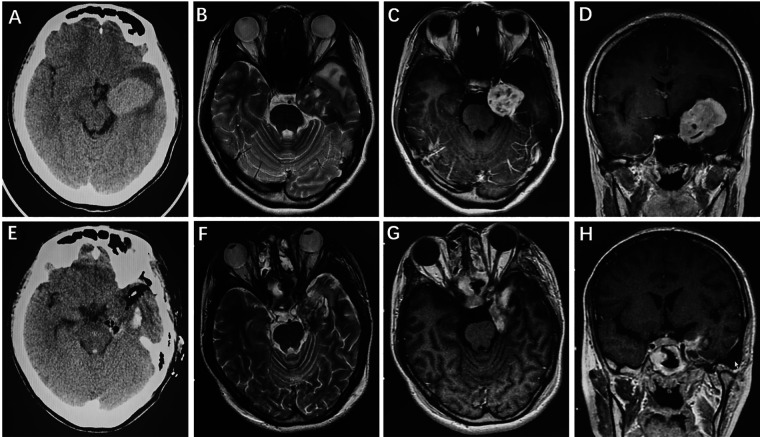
HPC invading the left CS and middle cranial fossa. Preoperative axial CT showed a slightly hyperintense lesion in the left CS and middle cranial fossa (A). Cranial axial MRI demonstrated isointensity on T2-weighted imaging (B). After enhancement, the axial and coronal MRI showed heterogeneous enhancement (C,D). Postoperative CT (E) and MRI (F–H) revealed that the tumor was subtotally removed.
